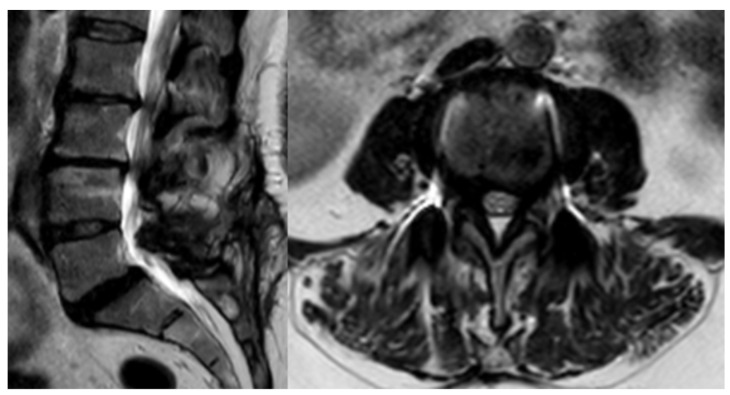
MR image of postoperative adjacent segment disease in a patient with L4-S1 rigid system.
